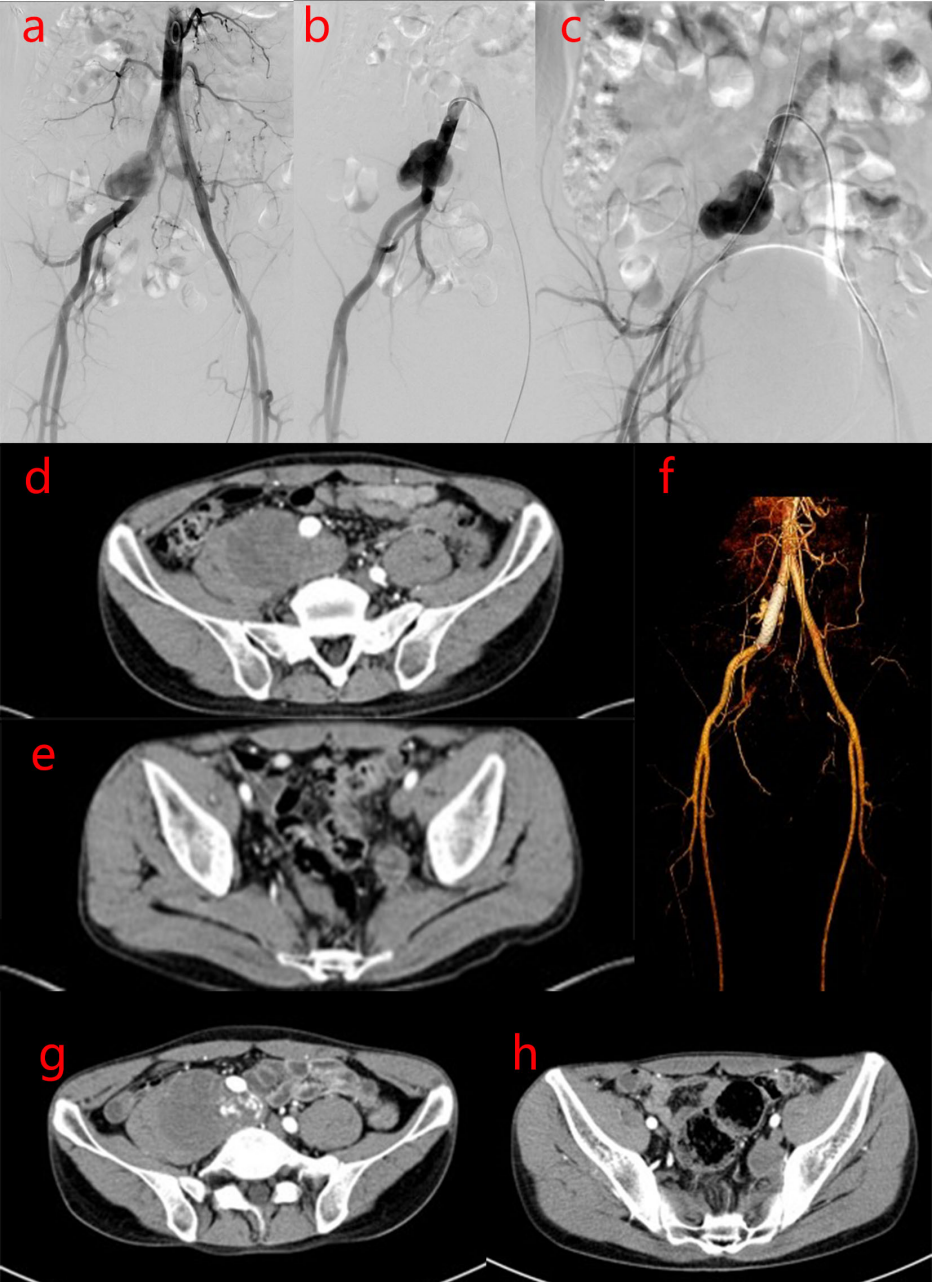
Imaging manifestations of pseudoaneurysm of the right CIA during bare stent implantation and after operation. (A–C). After bare stent implantation, DSA of the right inferior vena cava showed the following findings: the signal within the stent was unobstructed in the right CIA; a small amount of contrast agent at the stent in the right CIA still flowed into the cavity of the pseudoaneurysm and the size of the pseudoaneurysm became small; there was no contrast agent in the area of the left IIA pseudoaneurysm. (D–F). After 4 months of bare stent implantation, abdominal CTA showed that there is a small amount of contrast agent at the stent in the right CIA that continues to flow into the cavity of the pseudoaneurysm, leading to a slight increase in the size of the pseudoaneurysm. However, there was no contrast agent in the left IIA pseudoaneurysm area. (G, H). After 11 months of bare stent implantation, abdominal CTA showed a small amount of contrast agent at the stent in the right CIA that continued to flow into the cavity of the pseudoaneurysm, and the size of the pseudoaneurysm was similar to that of (D–F). However, there was no contrast agent in the left IIA pseudoaneurysm area.
